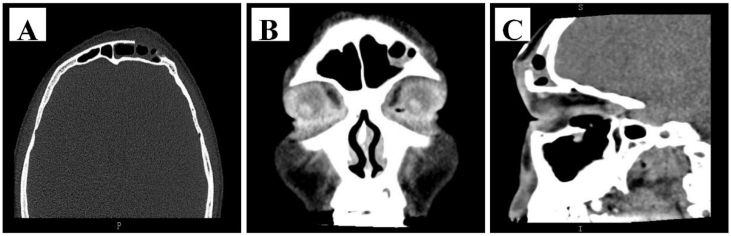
Postoperative sinus CT was performed: (A) Position of cross section; (B) Coronal section; (C) Sagittal section.
